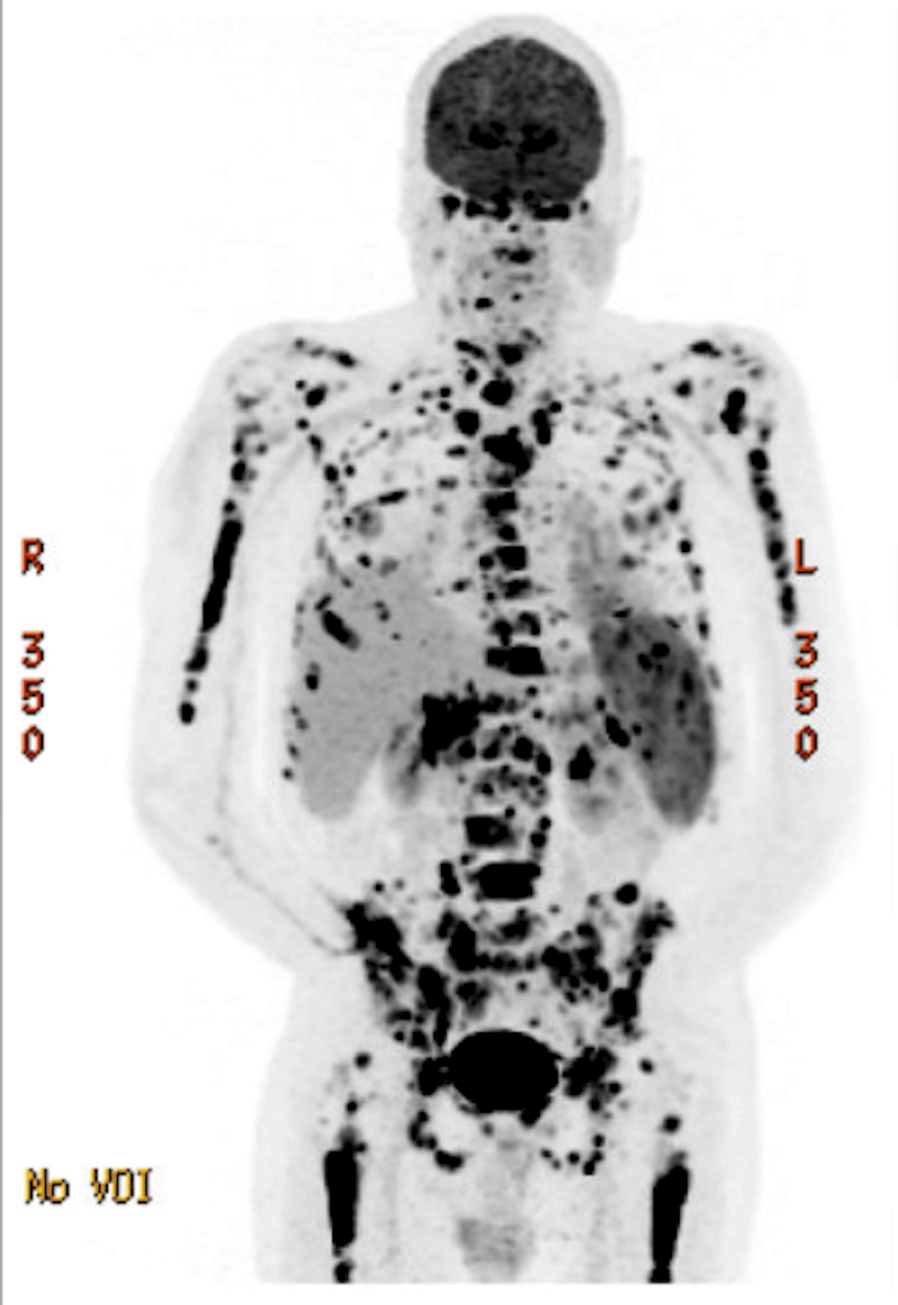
PET-CT findings before epcoritamab and pirtobrutinib combination therapy Findings are compatible with relapsed high-grade or transformed disease (5 PS = 5). Representative hypermetabolic right inguinal lymph node demonstrates a maximum SUV of 22.4 PET-CT: positron emission tomography-computed tomography; SUV: standard uptake variable
